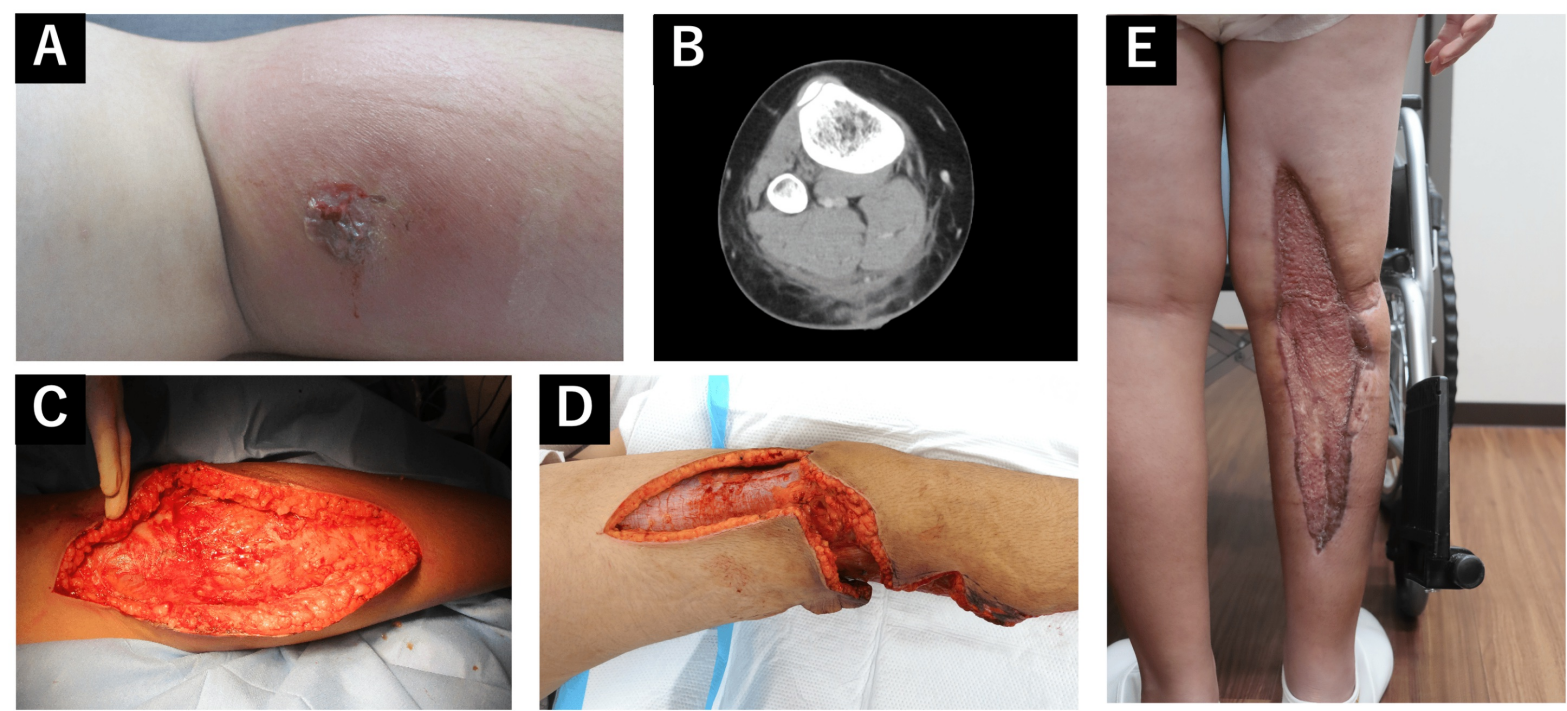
Clinical features of patient A: The patient’s right lower extremity on admission, showing swelling and erythema around the insect bite lesion located 5 cm distal to the popliteal fossa. B: Contrast-enhanced computed tomography (CT) scan of the right lower extremity demonstrating fat stranding and thickening of the superficial fascia (arrows), which are characteristic findings of necrotizing soft tissue infection. C: Intraoperative photograph of the wound bed after extensive surgical debridement, revealing necrotic fascia and subcutaneous tissue. D: An additional incision over the lateral femoral area. E: the wound after split-thickness skin grafting on day 21, demonstrating adequate graft take and progressive wound closure.
